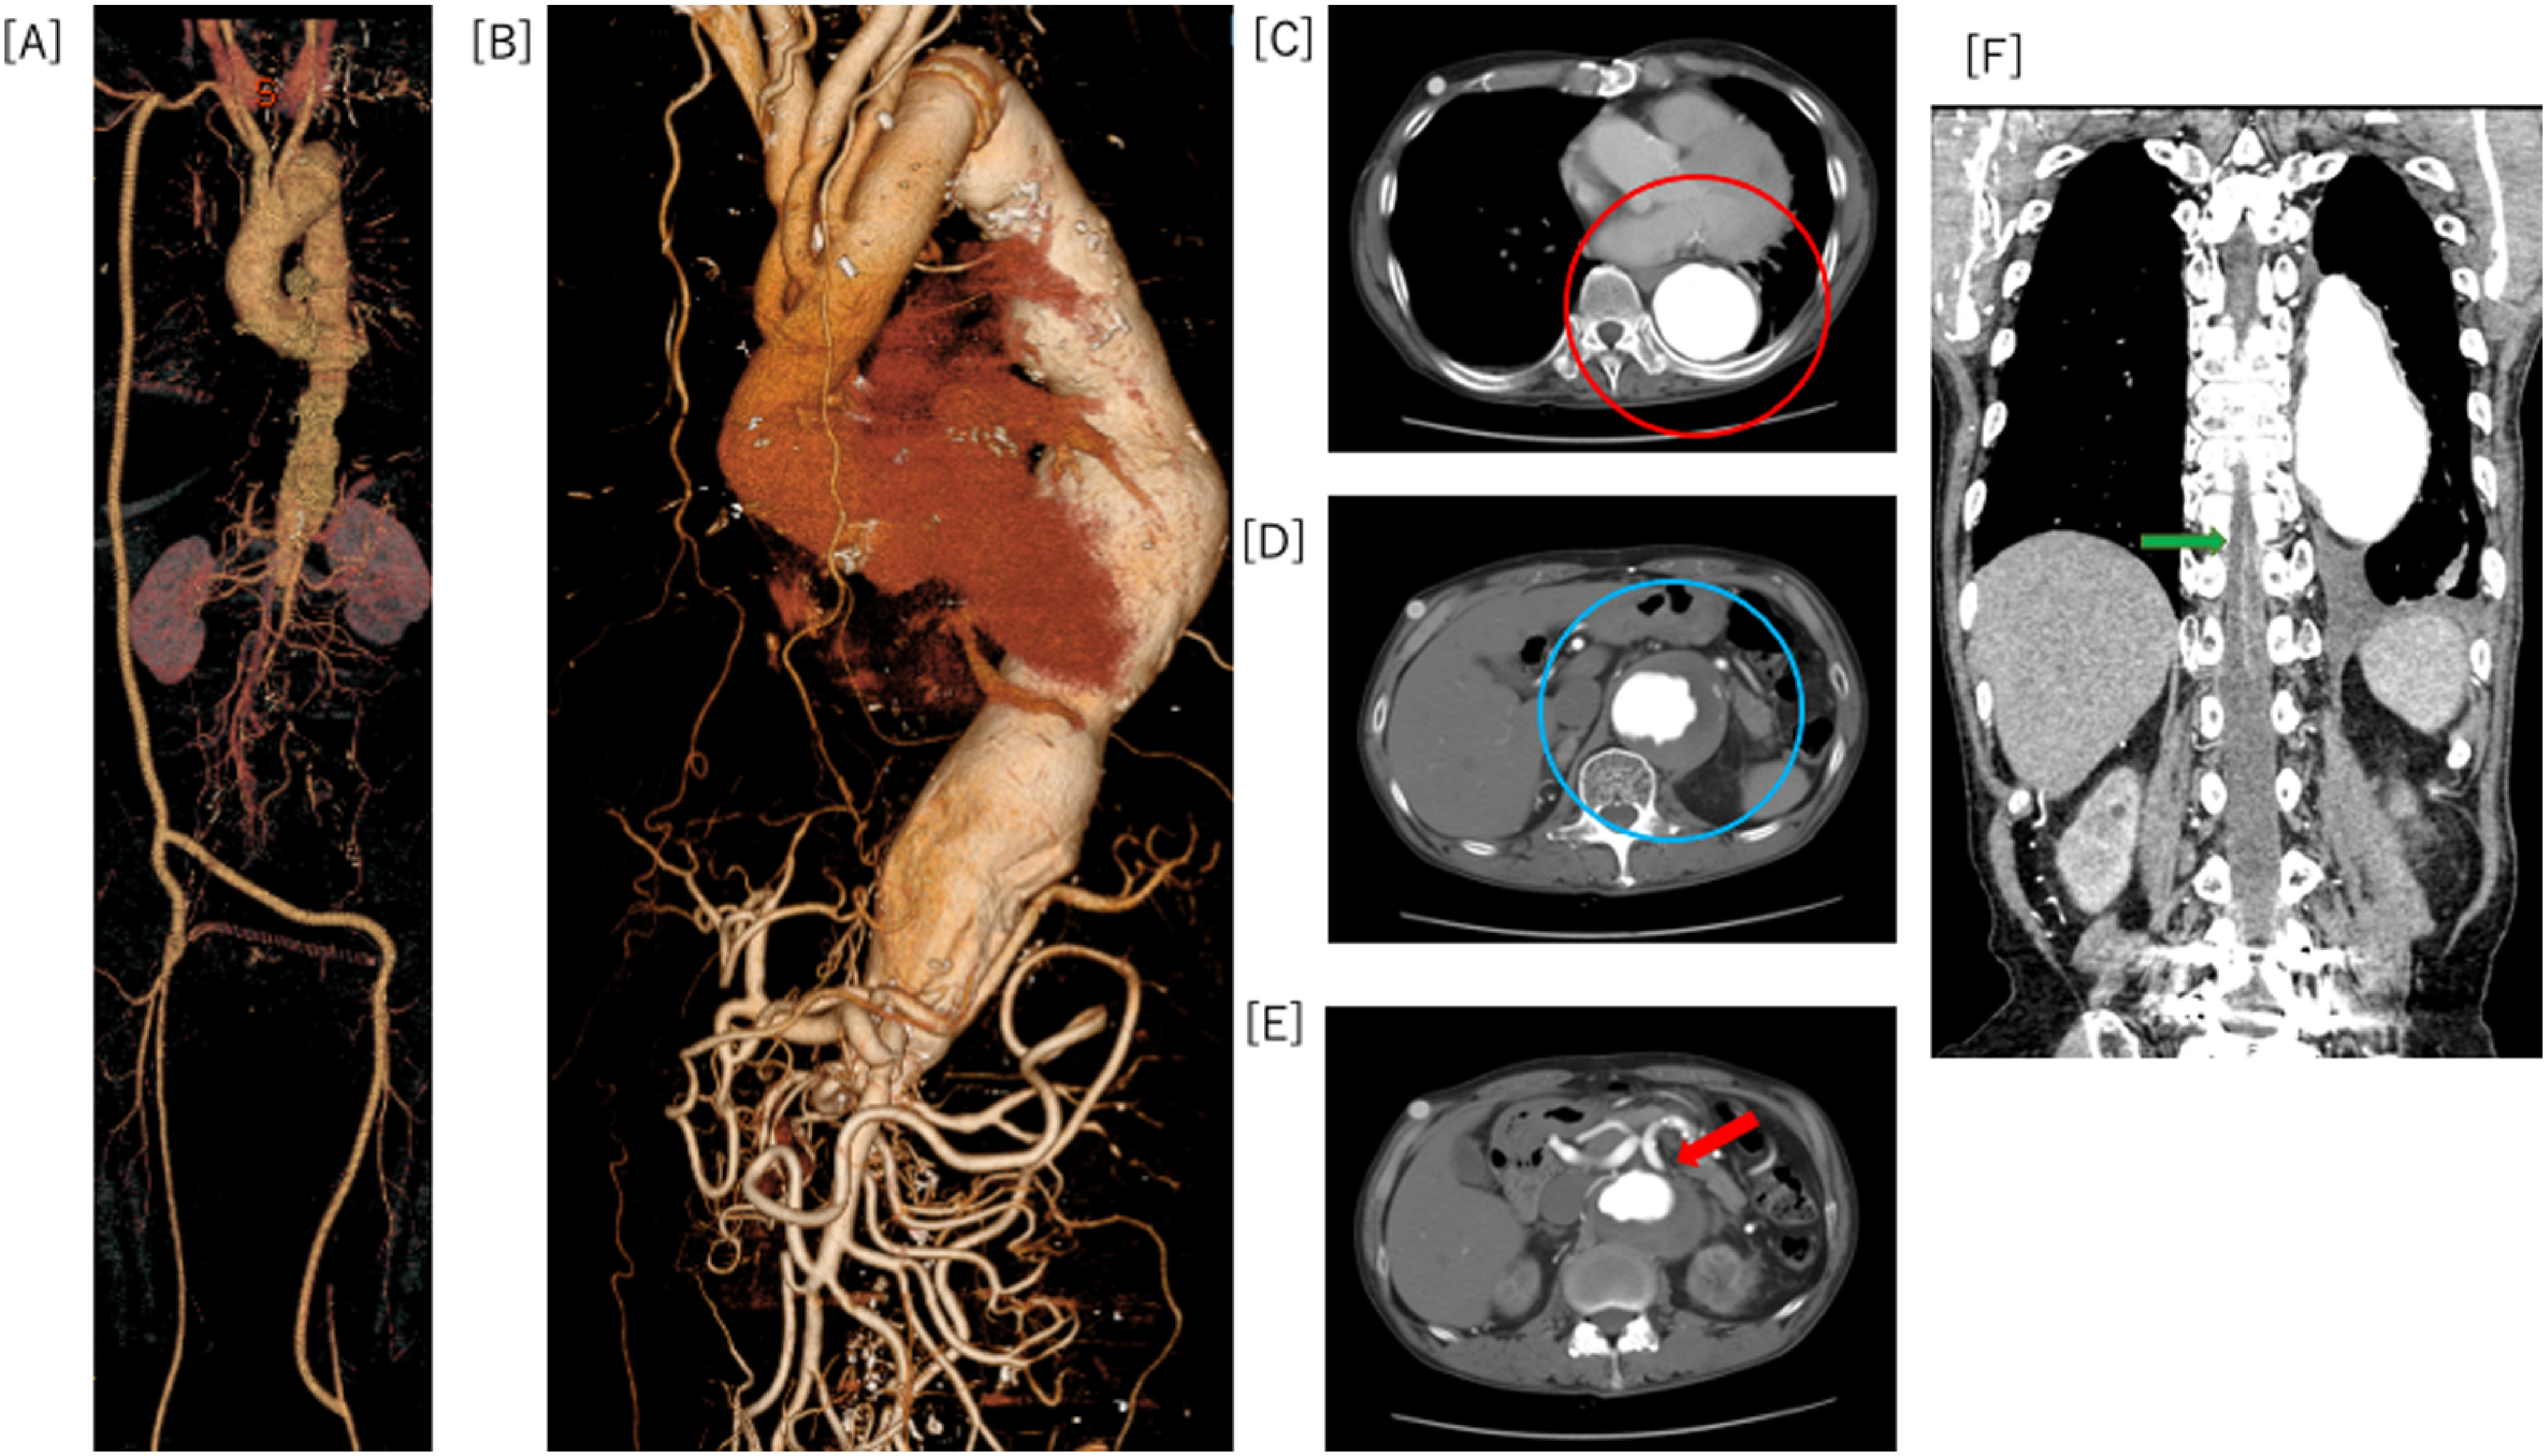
Fig. 1 Preoperative CT images.
